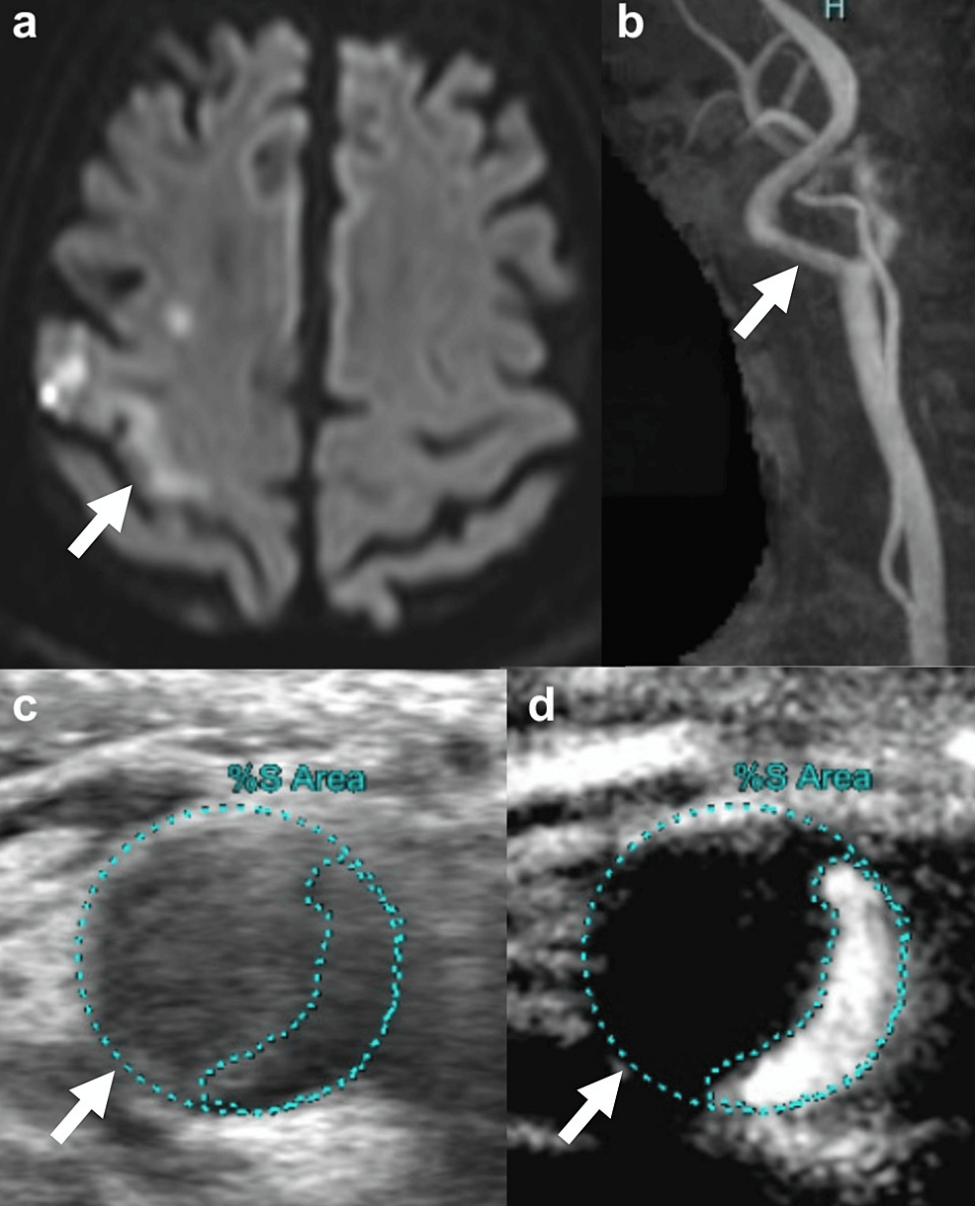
Preoperative MRI/MRA and carotid artery ultrasound (a) MRI showing hyperintensity on DWI in the right anterior central gyrus (white arrow). (b) MRA showing right ICA stenosis (white arrow). (c) Carotid artery ultrasound revealed low echoic plaque in the right ICA (white arrow). (d) Contrast-enhanced ultrasound showing a mobile plaque with stenosis (white arrow). DWI, diffusion-weighted imaging; ICA, internal carotid artery; MRA, magnetic resonance angiogram
